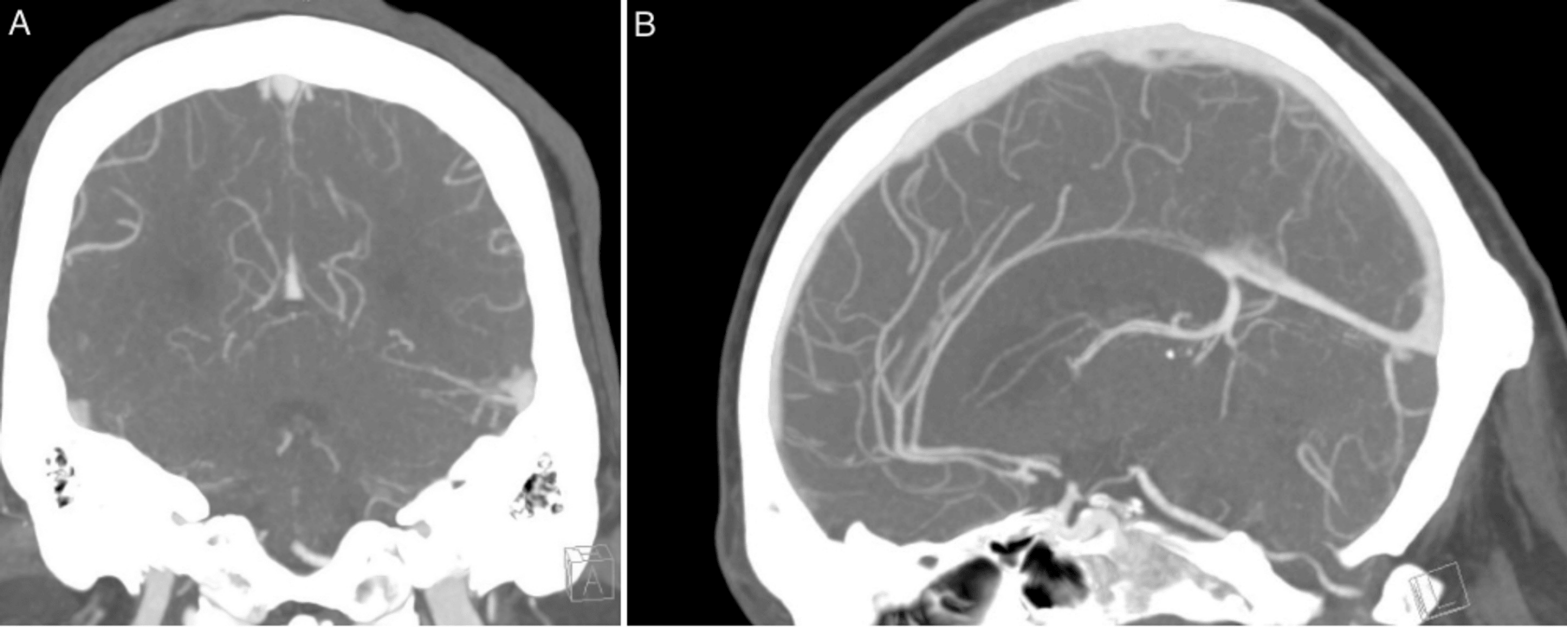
CT angiogram. A. Coronal view. B. Sagittal view. No abnormalities were detected on CT angiogram.
